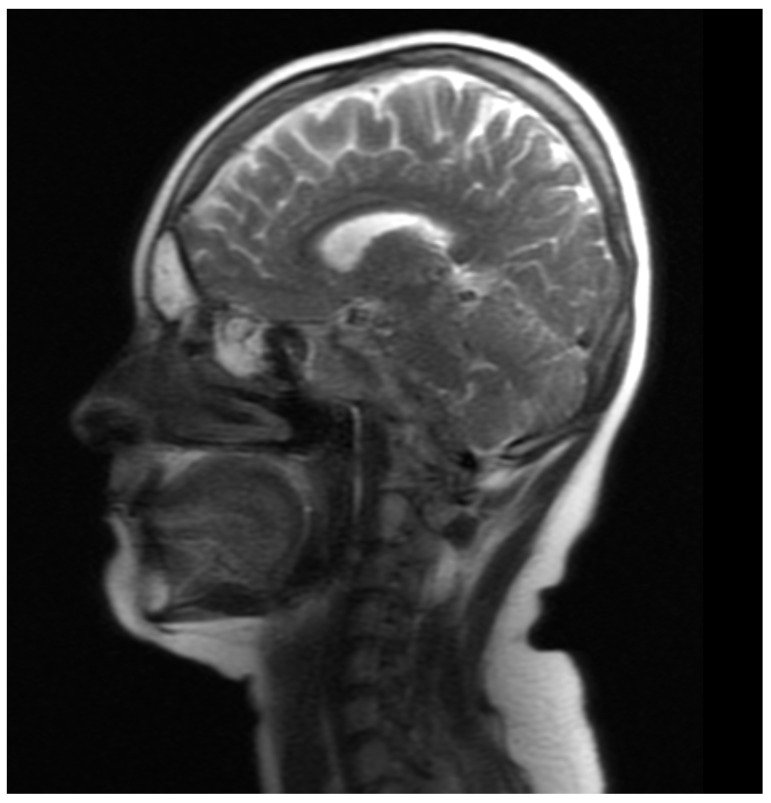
MRI of the head (sagittal plane) revealed frontal and ethmoid sinus involvement.
